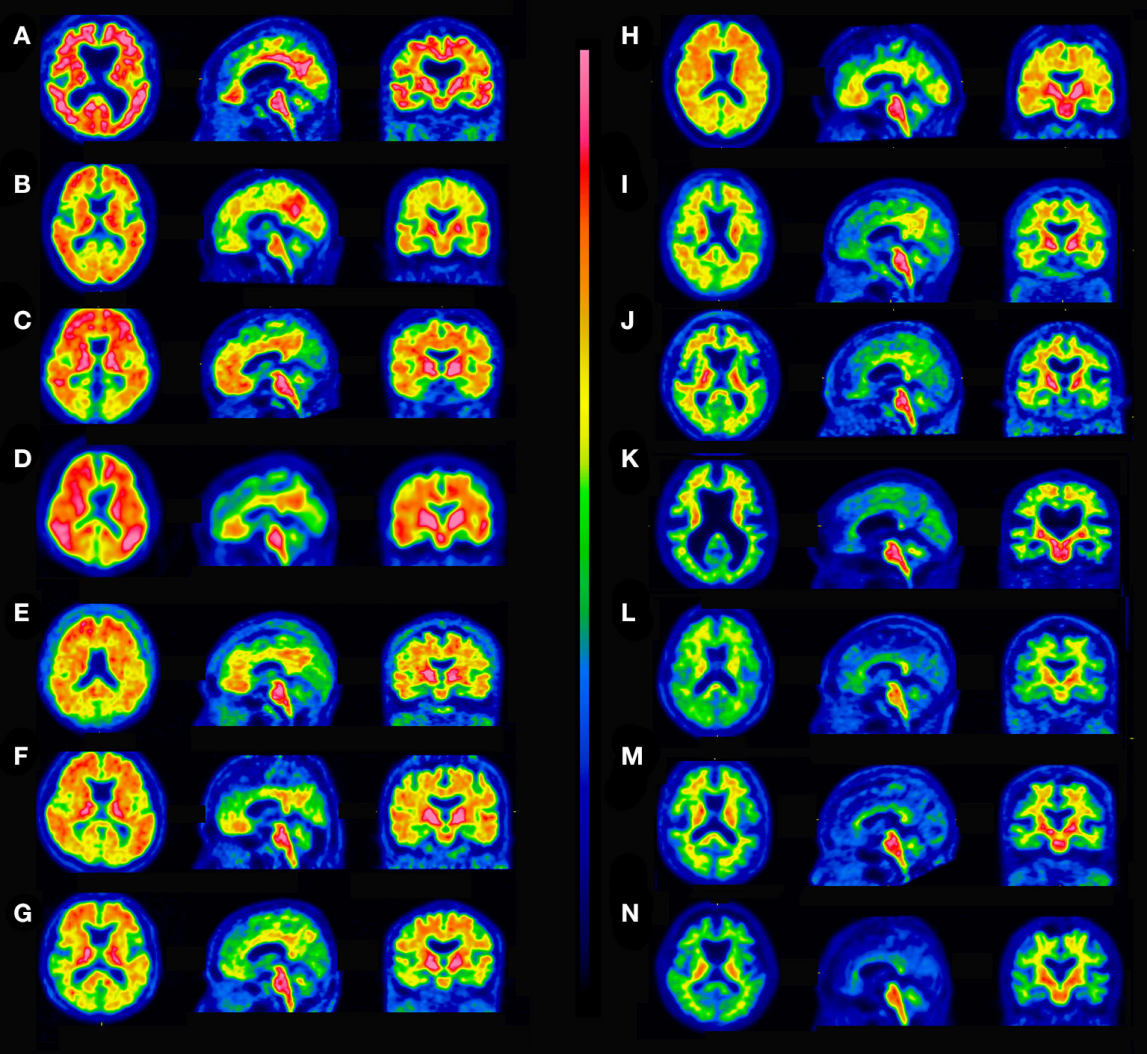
Concordant cases between quantitative assessments and visual interpretation. (A) Visually positive, SUVR 1.85, CL 104. (B) Visually positive, SUVR 1.74, CL 91. (C) Visually positive, SUVR 1.65, CL 80. (D) Visually positive, SUVR 1.57, CL 70. (E) Visually positive, SUVR 1.50, CL 60. (F) Visually positive, SUVR 1.41, CL 51. (G) Visually positive, SUVR 1.35, CL 42. (H) Visually positive, SUVR 1.24, CL 29. (I) Visually positive, SUVR 1.19, CL 23. (J) Visually positive, SUVR 1.13, CL 16. (K) Visually negative, SUVR 1.08, CL 12. (L) Visually negative, SUVR 1.04, CL 5. (M) Visually negative, SUVR 0.99, CL 0. (N) Visually negative, SUVR 0.90, CL −12.
